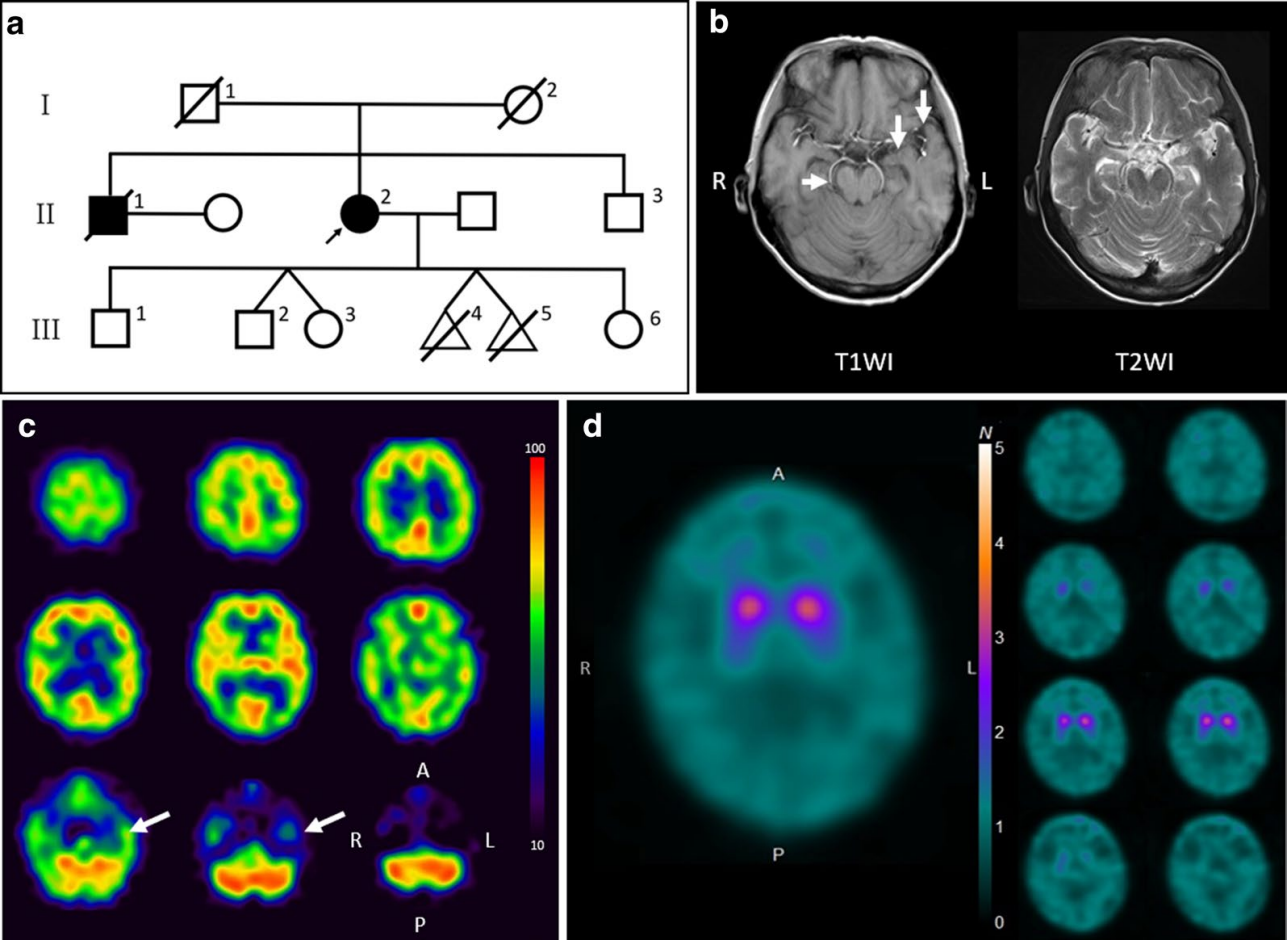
Clinical overview of the patients who presented with parkinsonism and progressive respiratory failure. a Our patient is shown as the proband (II-2) with an arrow. Patients with parkinsonism are shown as a filled circle or square for the proband and her elder brother (II-1), respectively. Her fourth (III-4) and fifth (III-5) children were conjoined twins and aborted before being delivered. Oblique lines indicate death. b T1- and T2-weighted brain magnetic resonance images (Siemens Magnetom Skyra 3.0 T) show mild atrophy in the midbrain, hippocampus, and temporal lobes, where marked with arrows. c I^123^-IMP brain single photon emission computed tomography indicates decreased blood flow in the anterior and medial regions of the left temporal lobes as marked with arrows. d The radioactivity in the bilateral putamen of dopamine transporter scintigraphy is depleted and is depicted as dot-like shapes (the specific binding ratio values using the Tossici-Bolt method were 1.72 for the right striatum and 1.62 for the left striatum). Abbreviations: T1WI, T1-weighted brain magnetic resonance images; T2WI, T2-weighted brain magnetic resonance images; A, anterior; P, posterior; R, right; L, left
